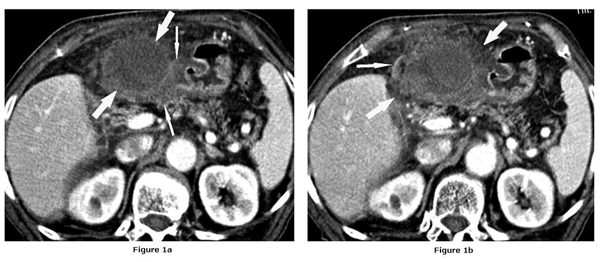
Contrast enhanced computed tomographic images show; loss of integrity of gallbladder wall (arrows). Diffuse thickening of the gastric antral wall (thin arrows). (b) CT image obtained upper level showed fluid collection (arrows) and well-enhanced curvilinear structure on the right side of the GB indicating cystic duct located on the right side of the gallbladder (thin arrow).
